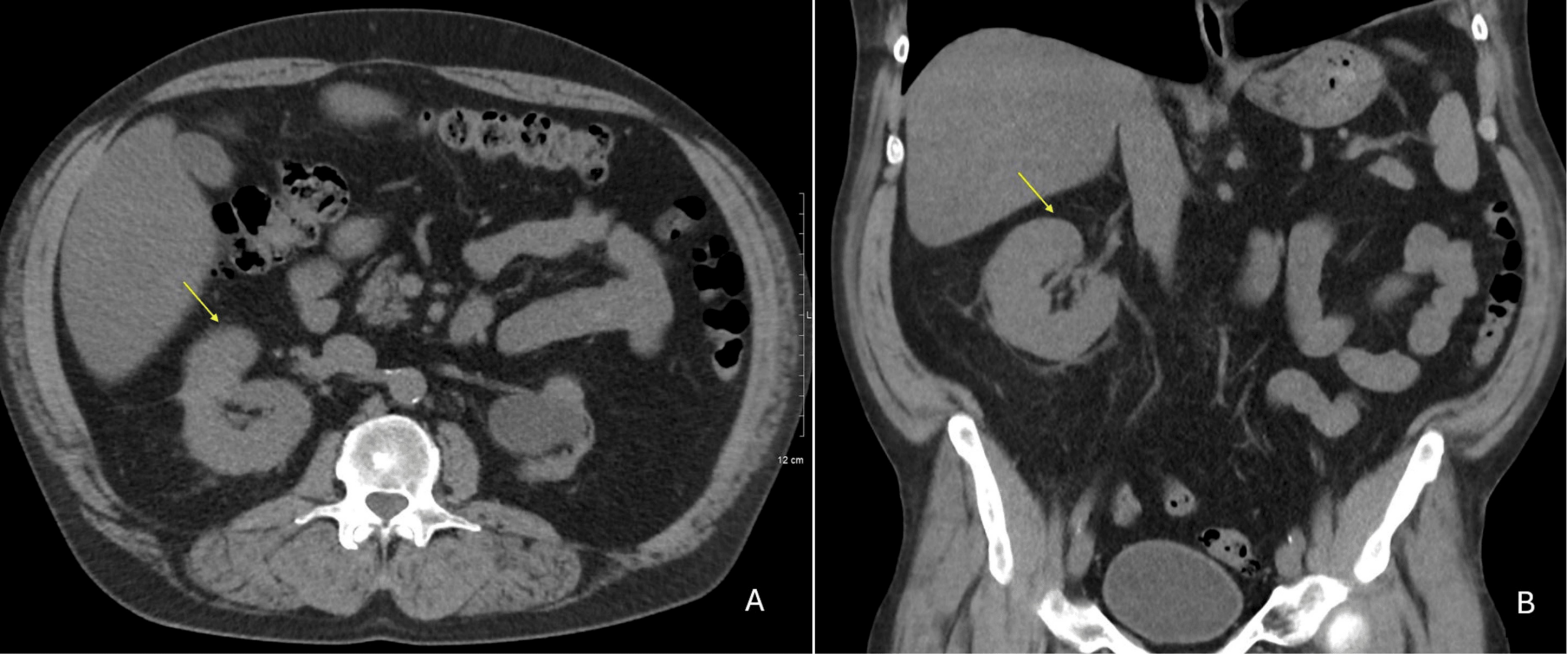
Noncontrast CT A/P S/P ablation of right kidney, axial (image A) and noncontrast CT A/P S/P ablation of right kidney, coronal Noncontrast CT abdomen/pelvis (A/P) status post (s/p) ablation of the right kidney around five years after microwave ablation. The mass is difficult to visualize on coronal (image B, yellow arrows) but can be seen on axial (image A, yellow arrows). Mass size decreased from 3.4 cm to 2.5 cm and has been stable in size.
